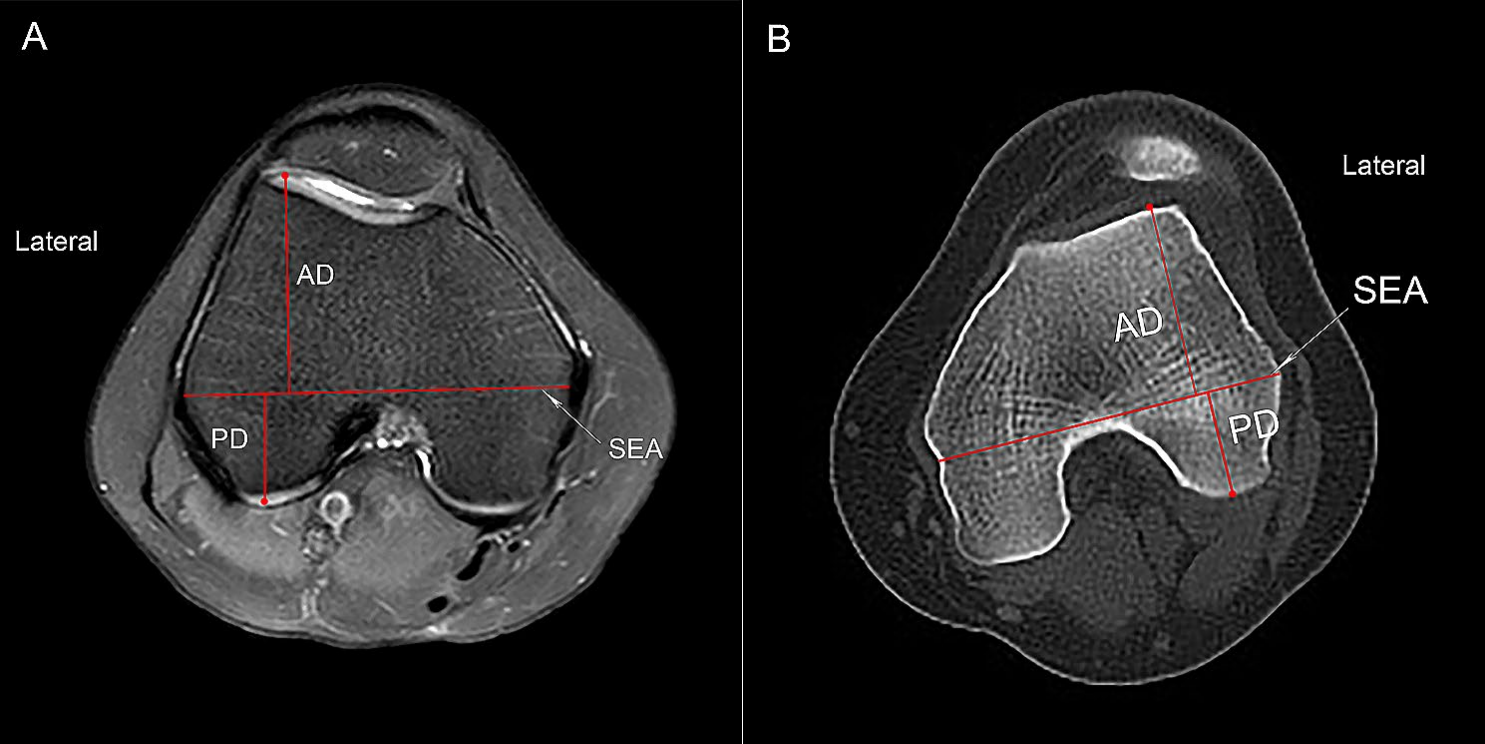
The measurement of lateral femoral condyle distance (LFCD). (A) The axial MRI slice showing the complete femoral trochlea with cartilage and intact posterior femoral condyles is selected. The surgical transepicondylar axis (SEA) are shown. The distance between the SEA and the posterior cartilaginous margin of the lateral condyle (PD) and the distance between the SEA and the anterior cartilaginous margin of the lateral condyle (AD) are measured. LFCD is defined as the ratio of PD/AD. (B) Similarly, the axial CT slice is selected. The length of anterior and posterior femoral condyles is measured to calculate LFCD
